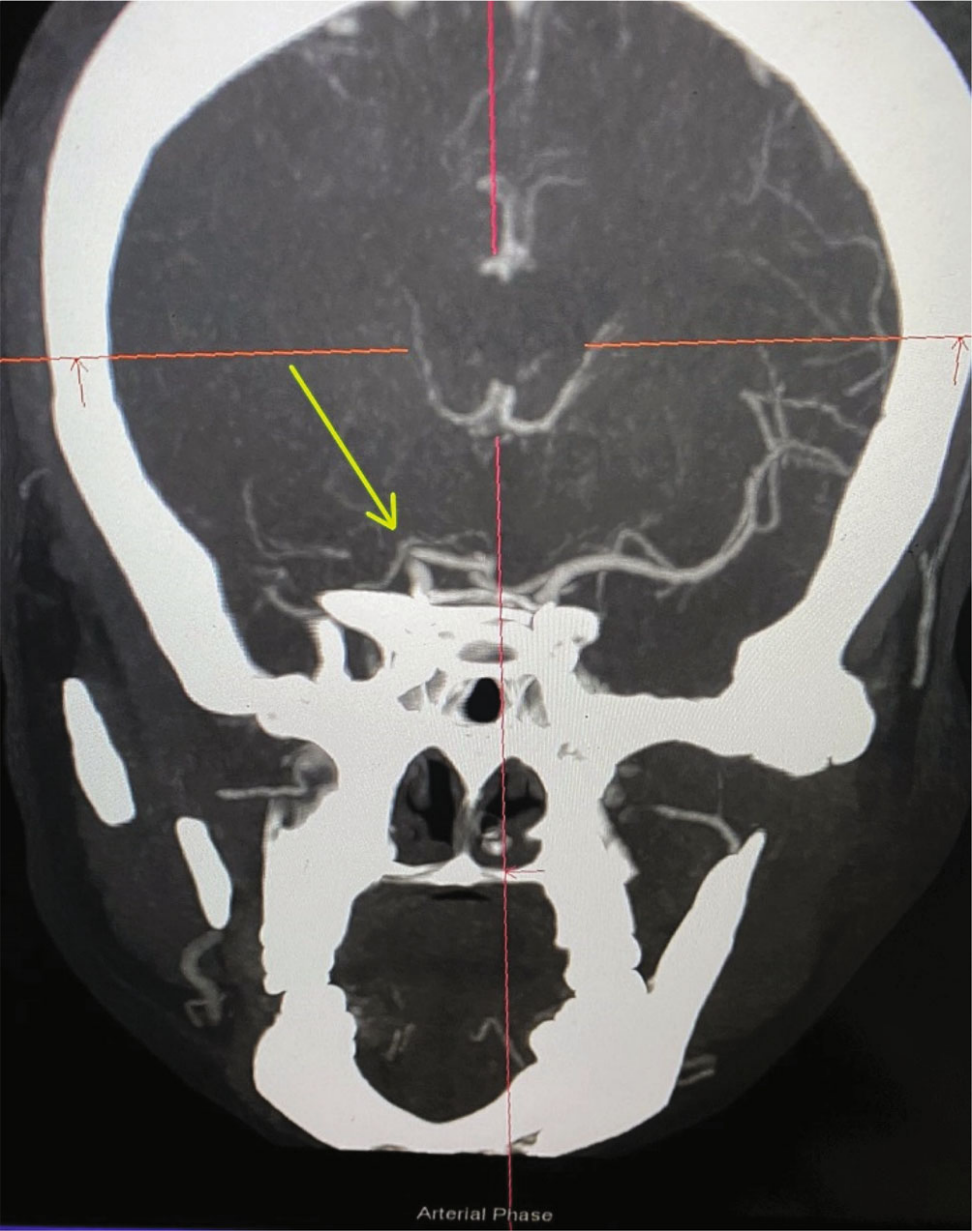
Cerebral CT angiography showing non-opacification of distal ICA and MCA (arrow).
